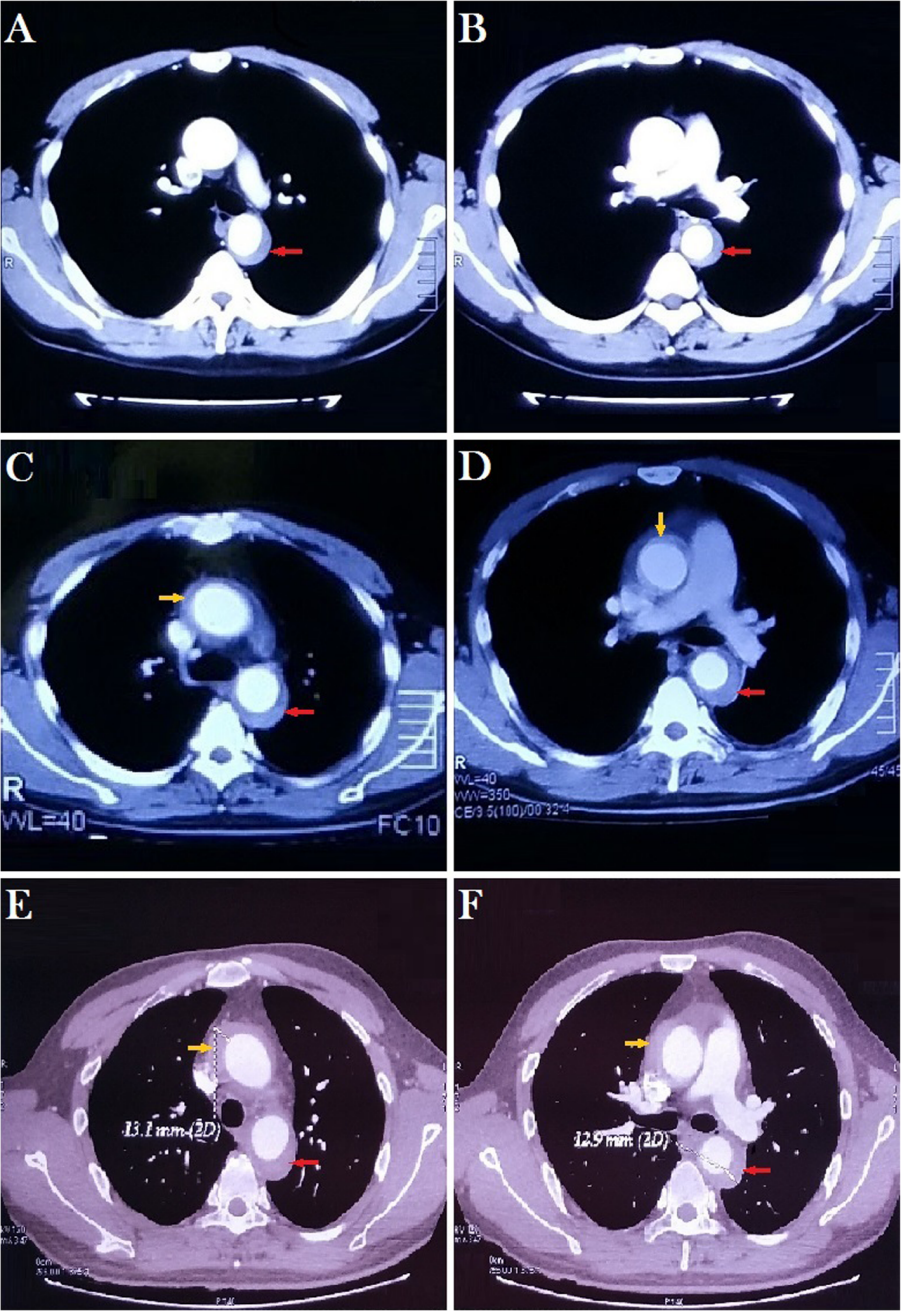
Preoperative enhanced computed tomography angiography (CTA) findings; (A, B): Presence of aortic dissection of the descending aorta on day 1 after disease onset (red arrow); (C, D): false lumen of ascending (yellow arrow) and descending aorta (red arrow) were seen on day 6; (E, F): Continuous thickening of false lumen in the ascending (yellow arrow) and descending aorta (red arrow) on day 12
